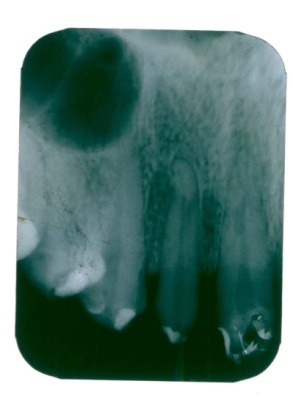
Periapical radiolucency and partial resorption of the interdental septum on 2.2 tooth
